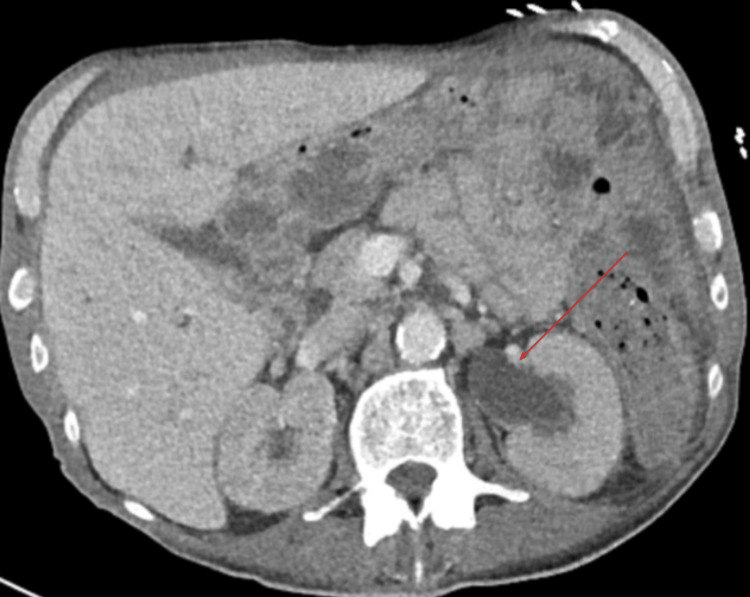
CT abdomen/pelvis in axial view demonstrating moderate to severe left hydronephrosis and hydroureter (red arrow) CT: Computed tomography
